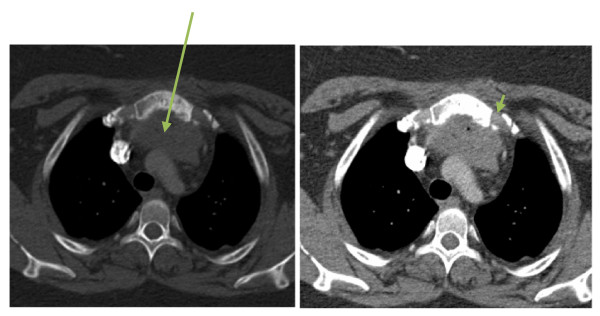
CT scan of chest. Mediastinal window: arrow shows retrosternal abscess behind the manubrium. Bone window: arrowhead showing cortical irregularity.
